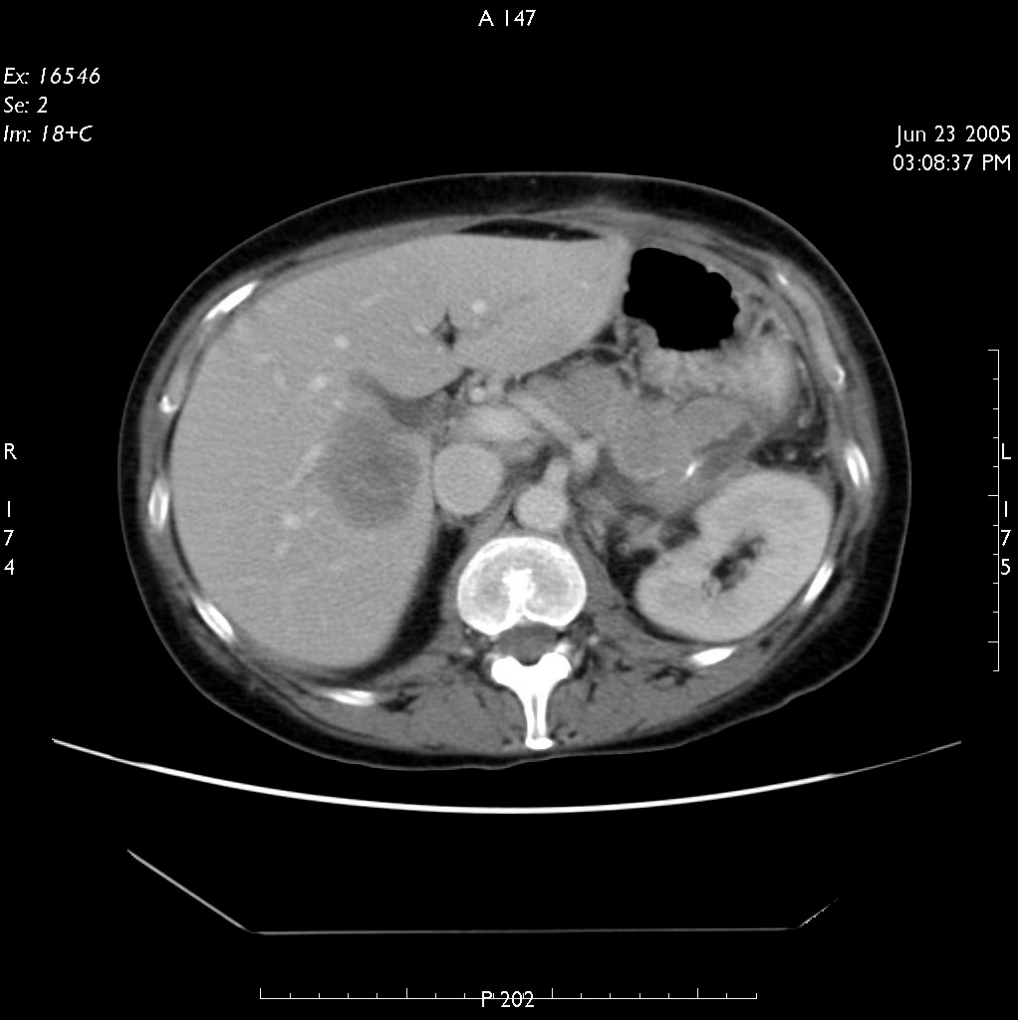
CT scan of abdomen showing liver metastases.
